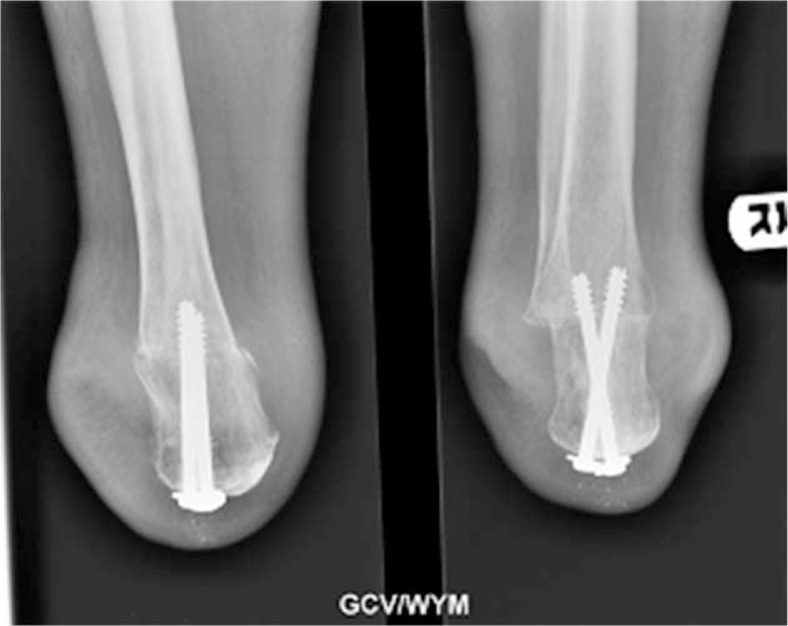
Radiographic views of a modified Pirogoff's stump at 6-month follow-up.
